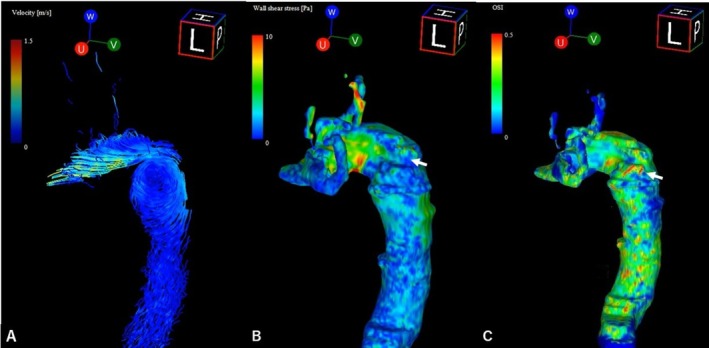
Four‐dimensional (4D) flow magnetic resonance imaging (MRI) analysis in Case 2. (A) Velocity streamlines visualize complex, vortical blood flow within the Kommerell's diverticulum (KD). (B) Wall shear stress (WSS) map shows diffusely low WSS. (C) Oscillatory shear index (OSI) map. The white arrow highlights a localized area of elevated OSI on the wall of the KD, indicating a potential hemodynamic risk zone.
